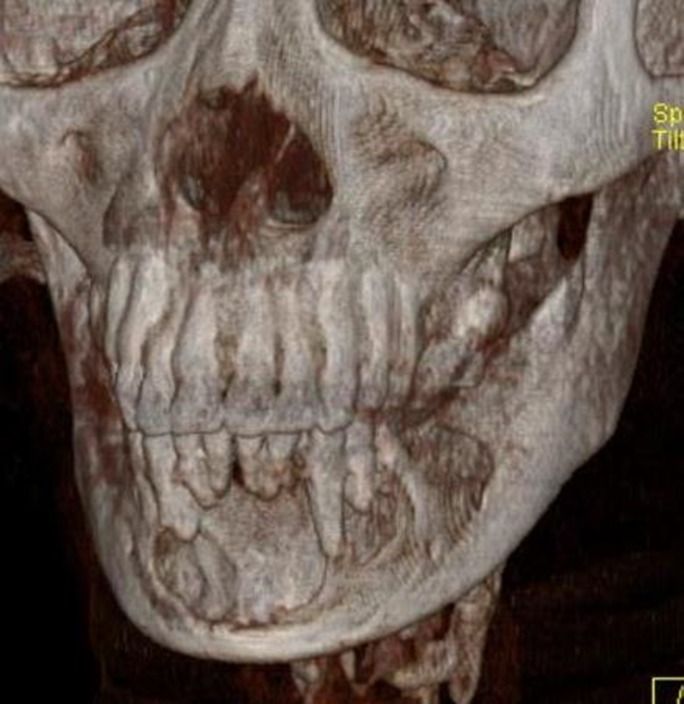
Panoramic radiography showed a well-defined multilocular radiolucency with thin corticated borders extending from the left side of the mandible from the tooth #36 region, crossing the midline up to tooth #44 on the right side. The internal structure was completely radiolucent. Root resorption of teeth #31, #32, #33, #34, #35, #41, #42, #43 and #44 was noted in the apical one-third (Figure 4).
